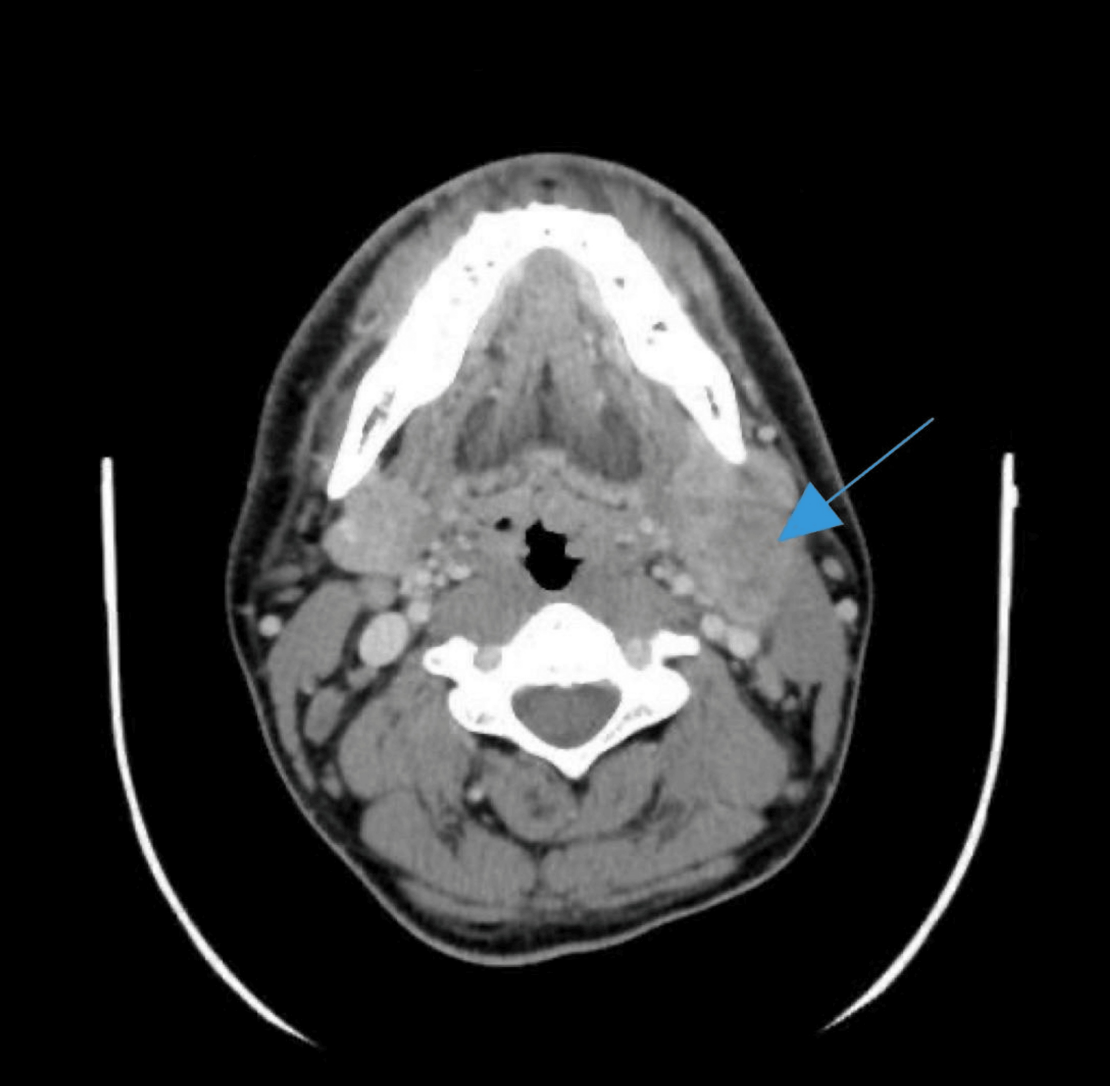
Axial computed tomography (CT) scan with contrast in the neck, demonstrating left level 2 lymph nodes with heterogenosity and central necrosis (blue arrow).
